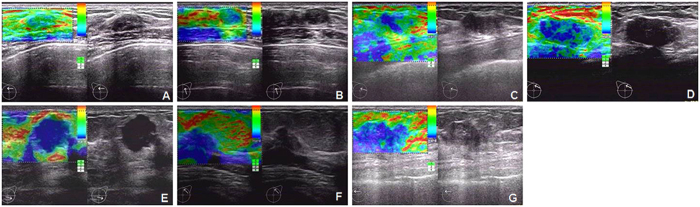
Representative pictures for the USE diagnosis (7-point elasticity scoring) of benign and malignant breast lesions. Scores <5 were classified as benign lesions, whereas scores ≥5 were classified as malignant lesions. (A) Elastosonography shows uniformed green (Score 1). Pathologic result: benign. (B) Elastosonography shows blue and green mosaic. Main color is green (Score 2). Pathologic result: benign. (C) Elastosonography shows center blue with green surrounding (Score 3). Pathologic result: benign. (D) Elastosonography shows blue and green mosaic. Main color is blue (Score 4). Pathologic result: benign. (E) Elastosonography shows lump was covered in blue area. (Score 5). Pathologic result: infiltrating ductal carcinoma. (F) Elastosonography shows lump its below was covered in blue area (Score 6). Pathologic result: infiltrating ductal carcinoma. (G) Elastosonography shows lump its surrounding was covered in blue area. Blue area is bigger than lump area (Score 7). Pathologic result: infiltrating ductal carcinoma.
